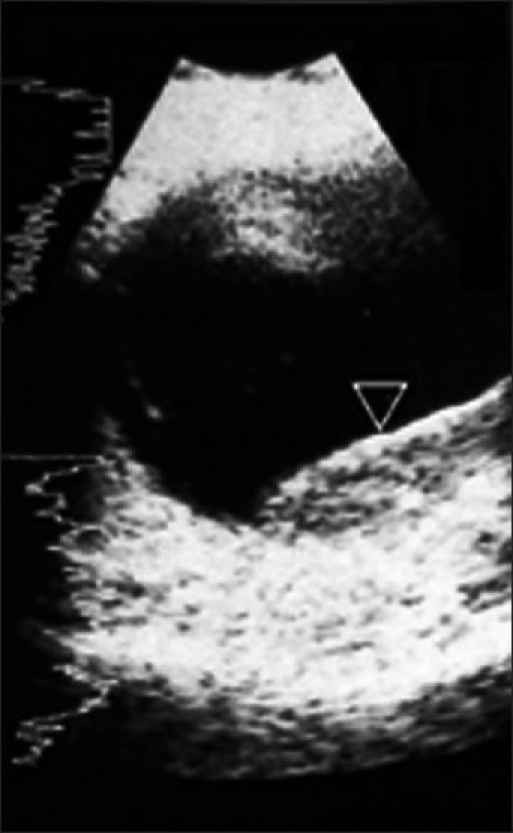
Ultrasonography of left eye shows a well-defined, echogenic lentiform-shaped lesion along the temporal aspect of the posterior coat of the globe associated with retinal detachment (►). A differential diagnosis of melanoma, metastasis was made
